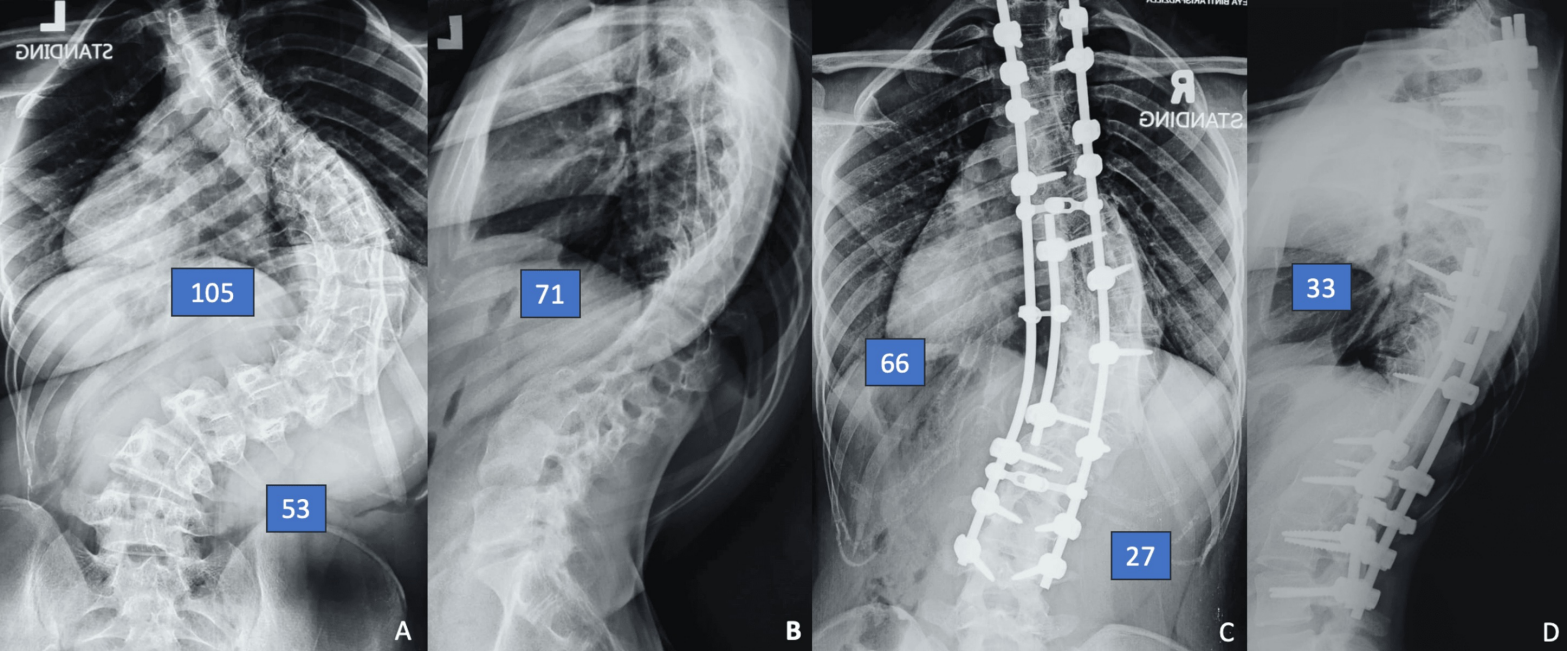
Whole spine radiograph of patient number 8. The pre-operative main thoracic (MT) Cobb angle of 105°, thoracolumbar/lumbar (TL/L) 53° (A) and thoracic kyphosis 71° (B). She underwent posterior instrumentation and fusion using triple-rod technique from T2-L4. The post-operative x-rays show coronal curve correction of MT to 66°, TL/L to 27° (C) and kyphosis correction to 33° (D). The details are provided in Table 2.
